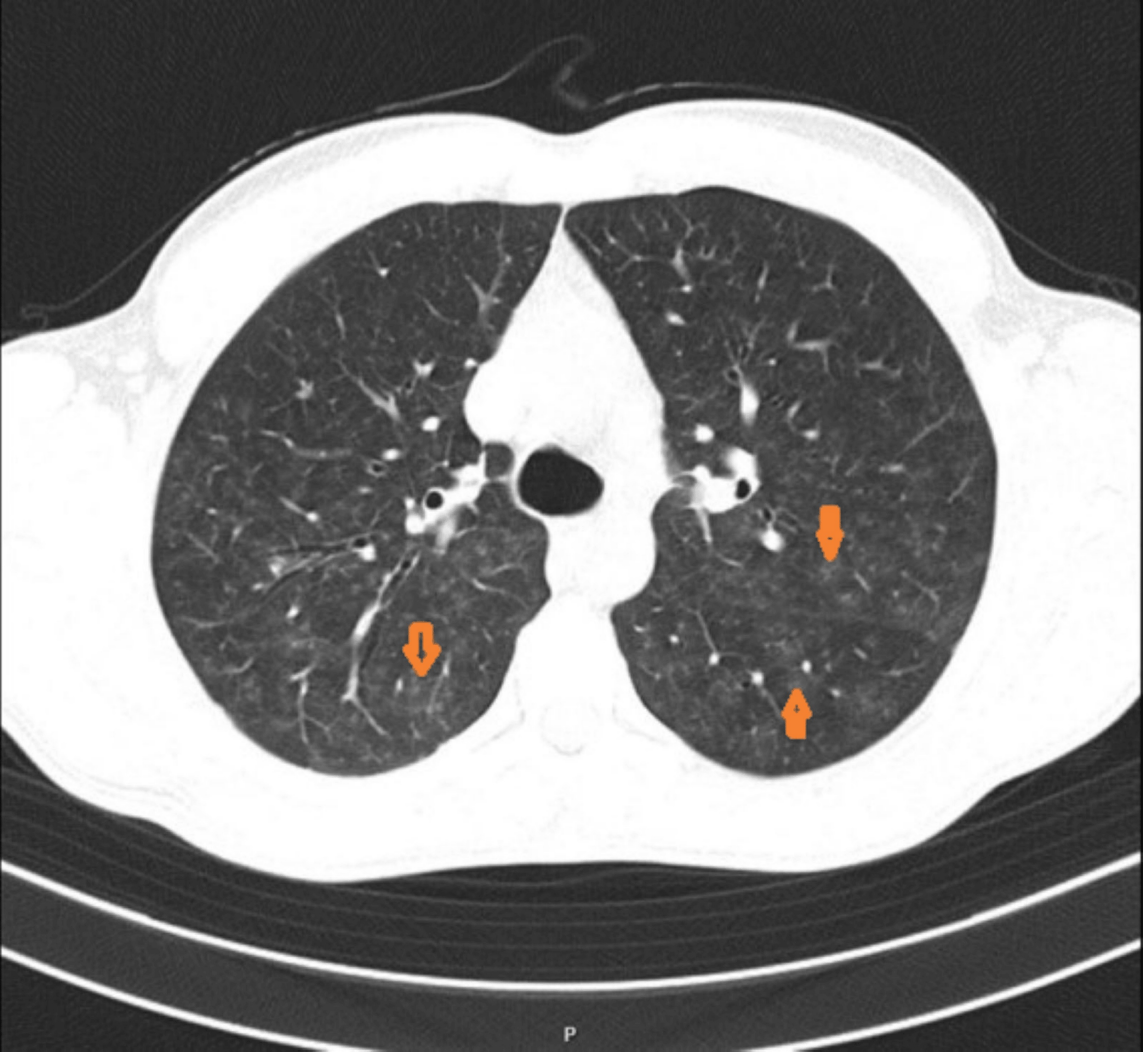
Chest computed tomography (CT) scan showing bilateral ground-glass opacities (orange arrows), consistent with alveolar hemorrhage.
